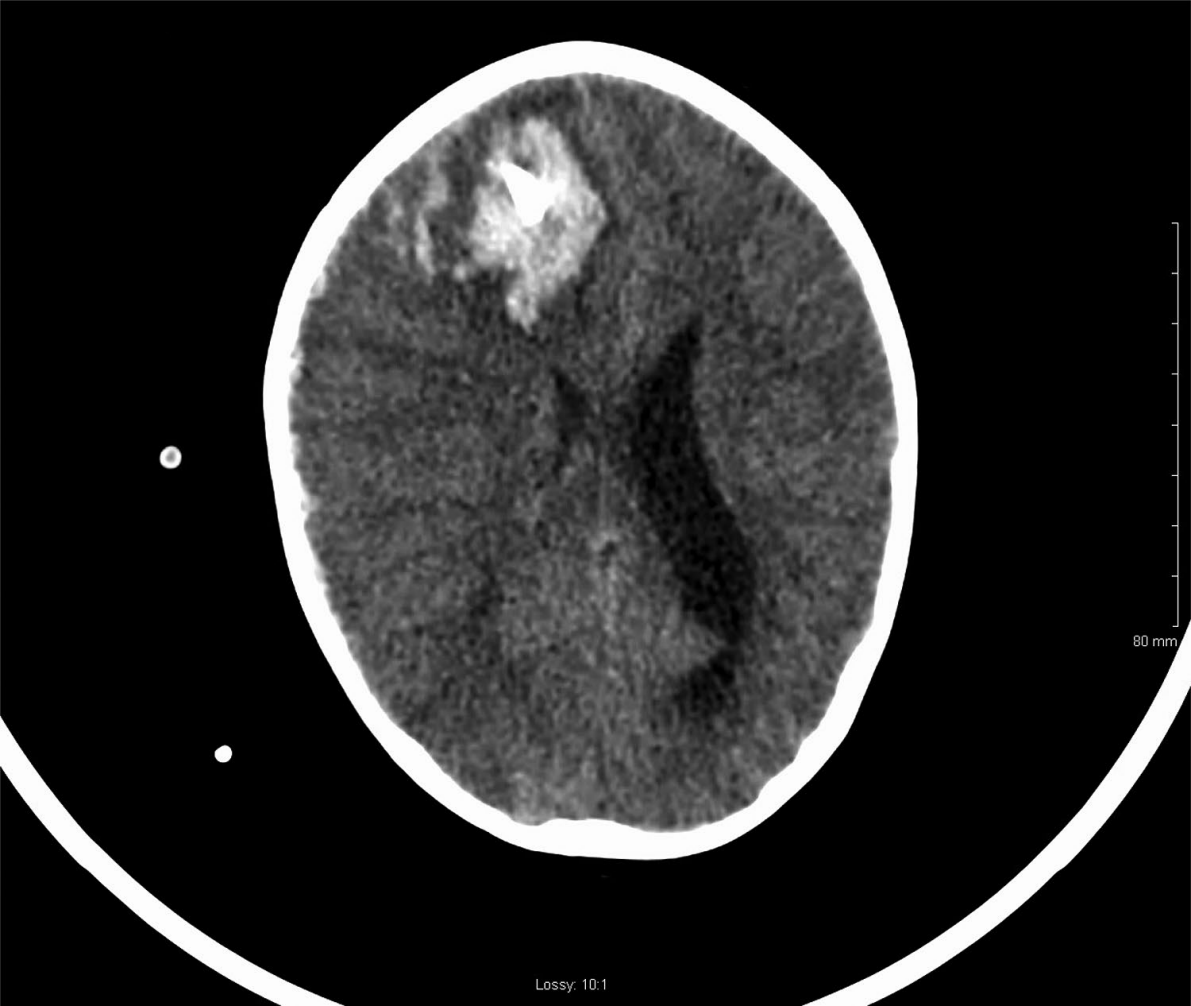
Postoperative CT brain (axial view) of spontaneous frontal haemorrhage
